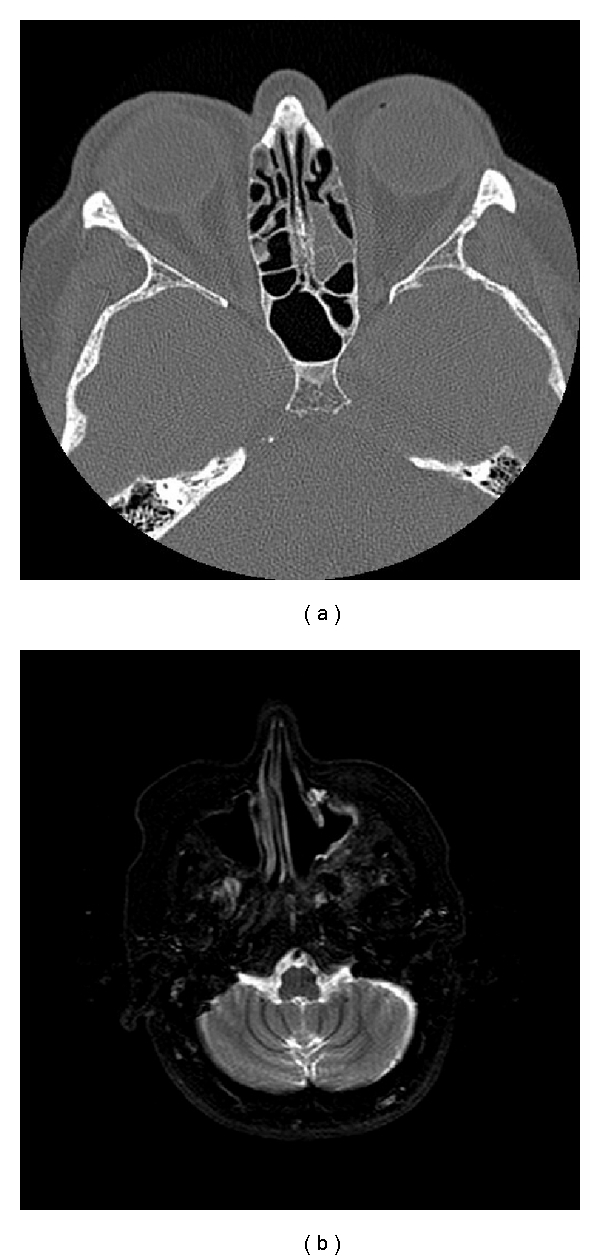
(a) CT scan taken in 2007 showing soft tissue with bony wall destruction in the patient's left posterior ethmoid sinus, which was a recurrence of the malignancy and treated with topical chemotherapy. (b) MRI scan taken in 2008 showing an area of high signal in the patient's left maxillary antrum, indicating the need for a biopsy to be performed.
